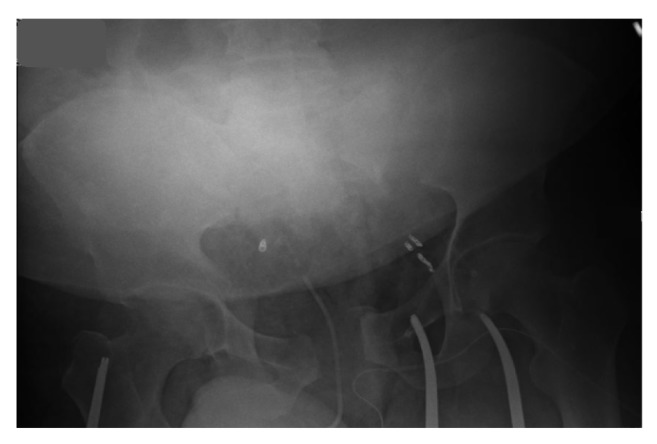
Portable radiograph of the pelvis. There is severe diastasis of the pubic symphysis, avulsion of the left superior pubic ramus, and separation of the left sacroiliac joint.
